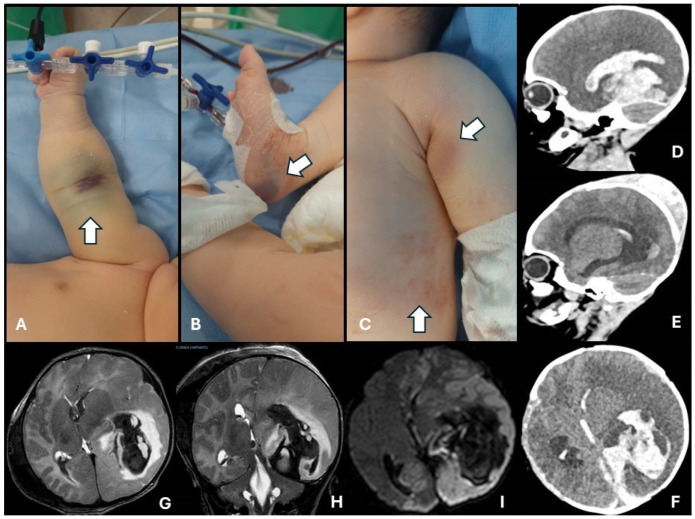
Extensive ecchymosis and edema at venous access sites (panel (A), arrow) and at the site of previous capillary sampling (panel (B), arrow); hematoma at infant’s holding site level (panel (C), arrows). CT scans (panels (D–F)) and MRI (T1-weighted coronal, panel (G); and transversal, panel (H), scans) of the extended tetraventicular hydrocephalus, originating from subdural, parenchymal, and intraventricular hemorrhage, with associated ischemic infarction and cytotoxic edema (panel (I), DWI-weighted MRI scan), interesting the left hemisphere, with significant compression on the surrounding brain structures.
